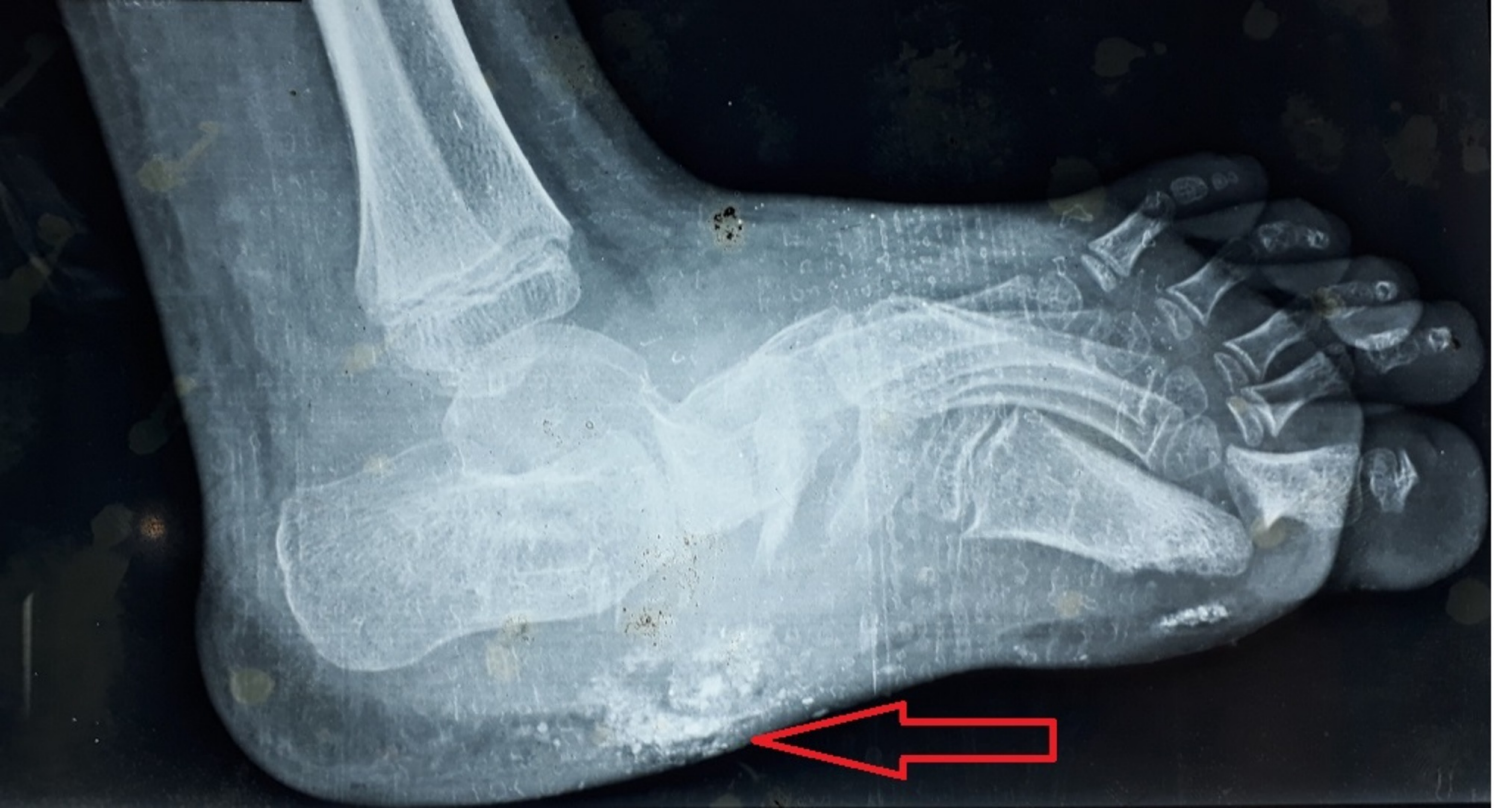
Magnetic resonance imaging (MRI) of the right foot showing lytic center with a ring of sclerosis in the tarsal bones
